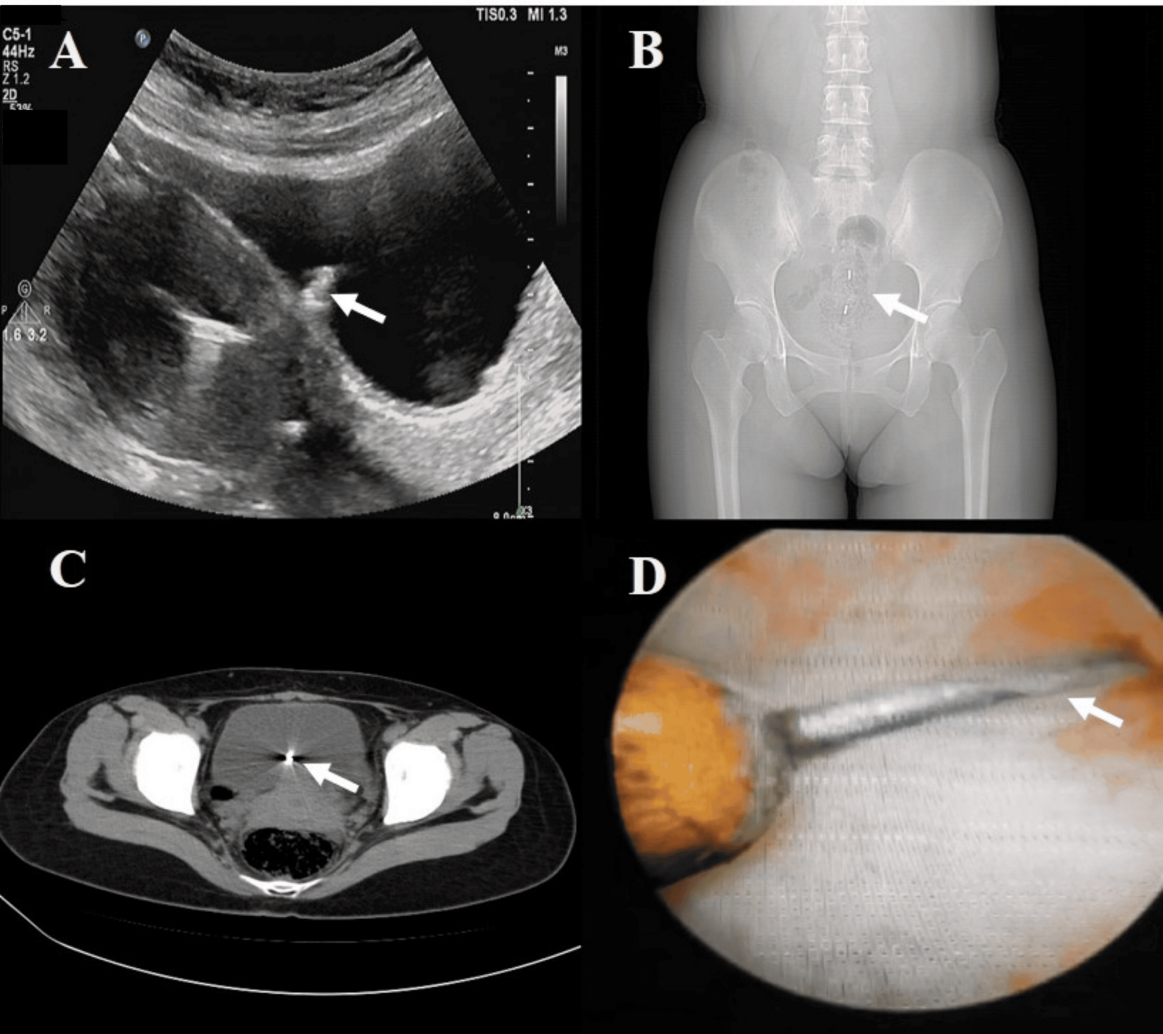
Preoperative examination: (A) Transabdominal color Doppler ultrasonography; (B) Pelvic plain film (prone position); (C) Abdominal and pelvic CT scan; (D) Cystoscopic examination.
